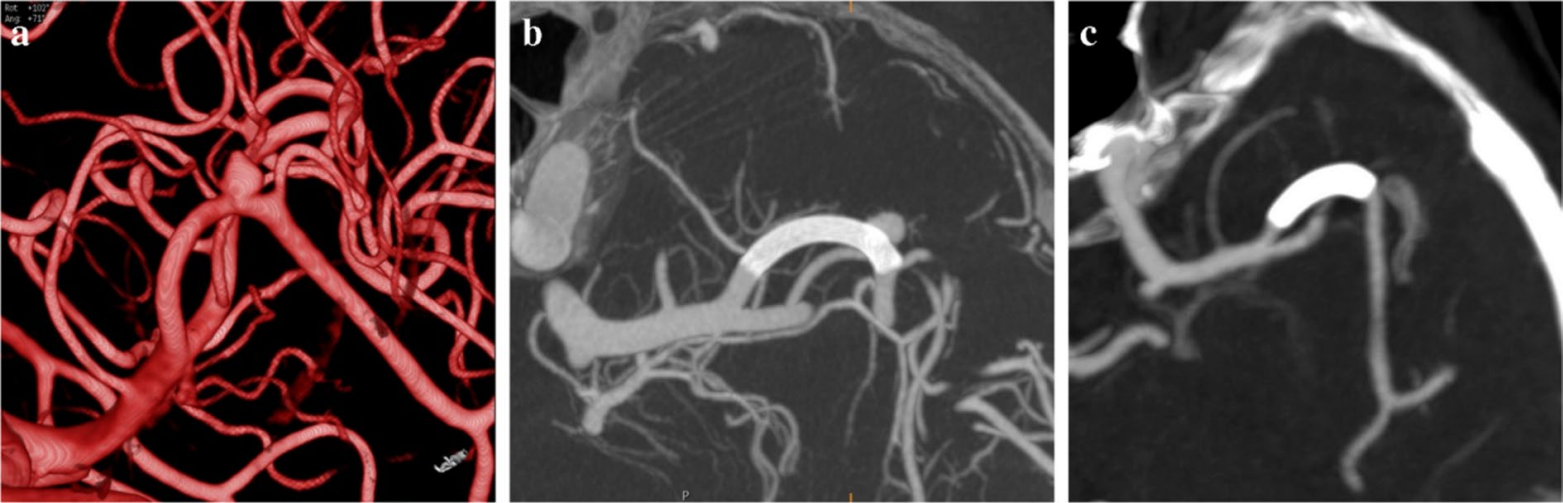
A 69-year-old female patient with a positive family history of cerebral aneurysm. (a) Axial 3D angiogram showing a saccular aneurysm located in the M2–3 segment of the left MCA. (b) Cone-beam CT obtained after SVB stent implantation. (c) Six-month follow-up CTA reveals disappearance of the aneurysm
